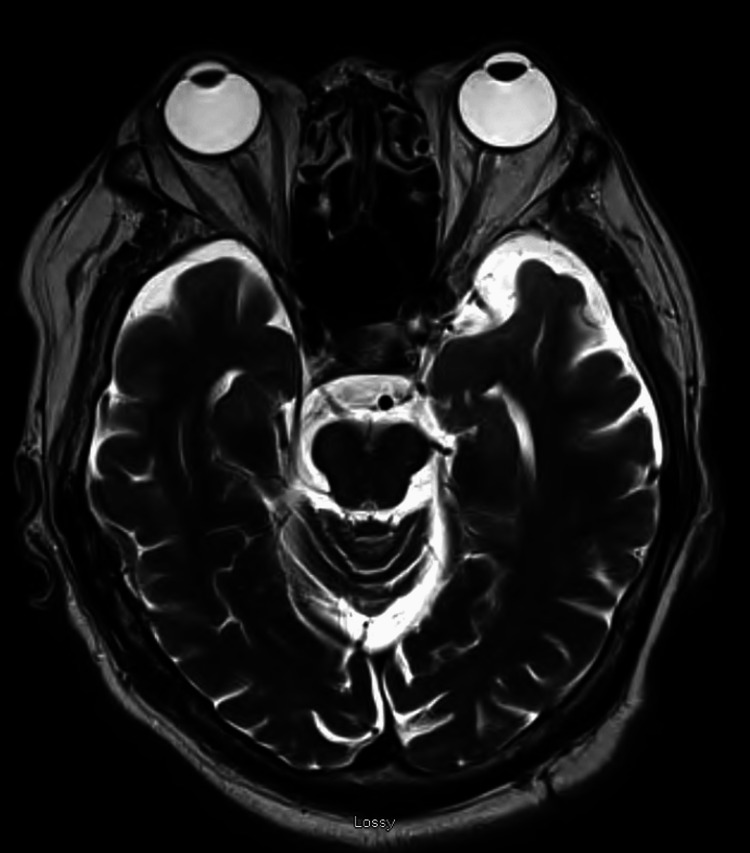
MRI of the brain demonstrating normal findings
